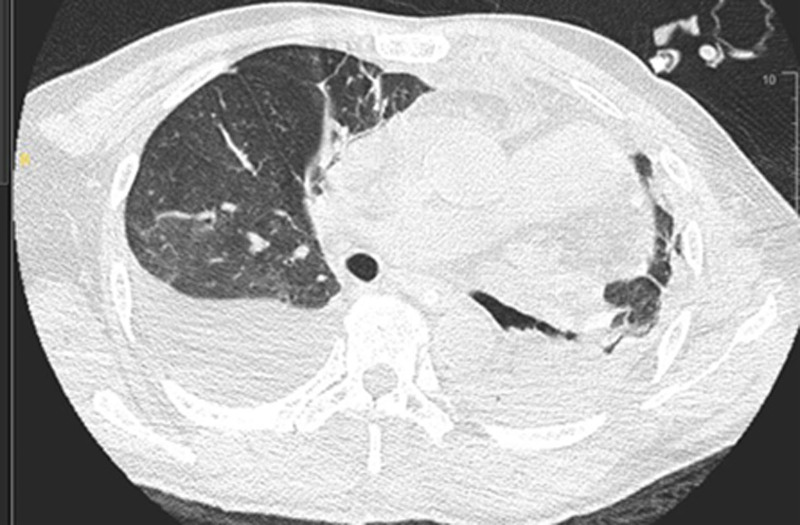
Postoperative day 3 computed tomography (CT) scan showing bilateral pleural effusions. CT = computed tomography.
